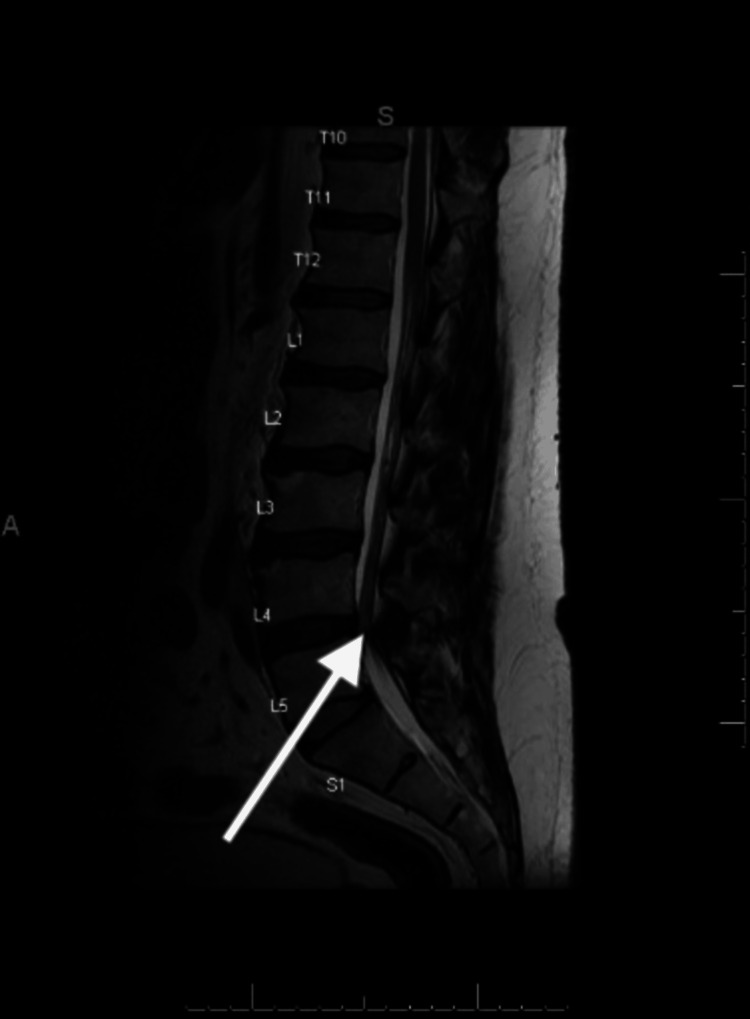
Sagittal MRI view of the lumbar spine for Case 2, with a white arrow pointing to spinal canal narrowing.
